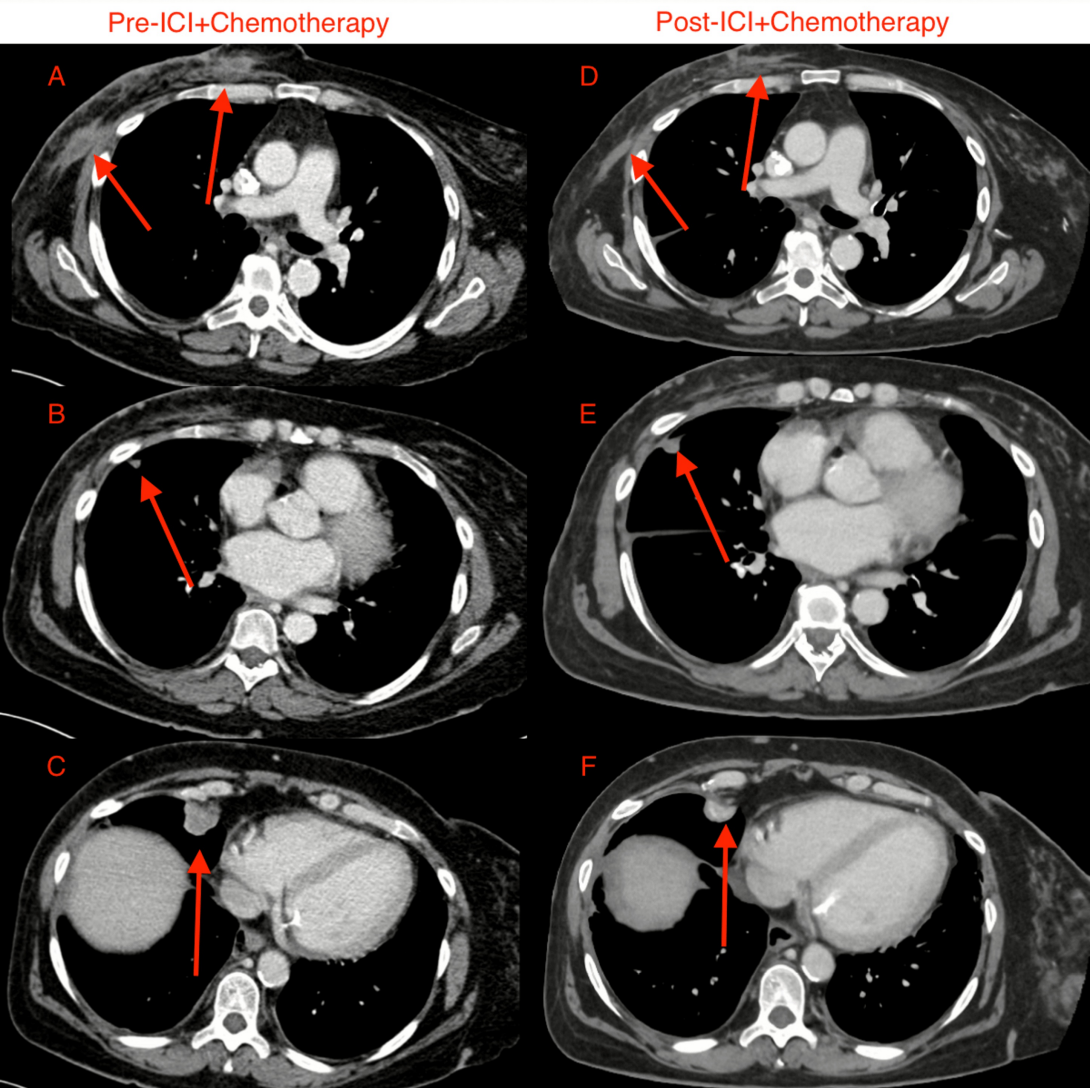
Metastatic foci seen on CT scan prior to and after initiation of ICI + Chemotherapy. (A) Two right chest wall lesions prior to treatment. (B) Right middle lobe juxta-fissural pulmonary nodule prior to treatment. (C) Right middle lobe pulmonary nodule prior to treatment. (D) Two right chest wall lesions after treatment. (E) Right middle lobe juxta-fissural pulmonary nodule after treatment. (F) Right middle lobe pulmonary nodule after treatment. The red arrow points to the lesion of interest in each image. ICT: immune checkpoint inhibitors.
